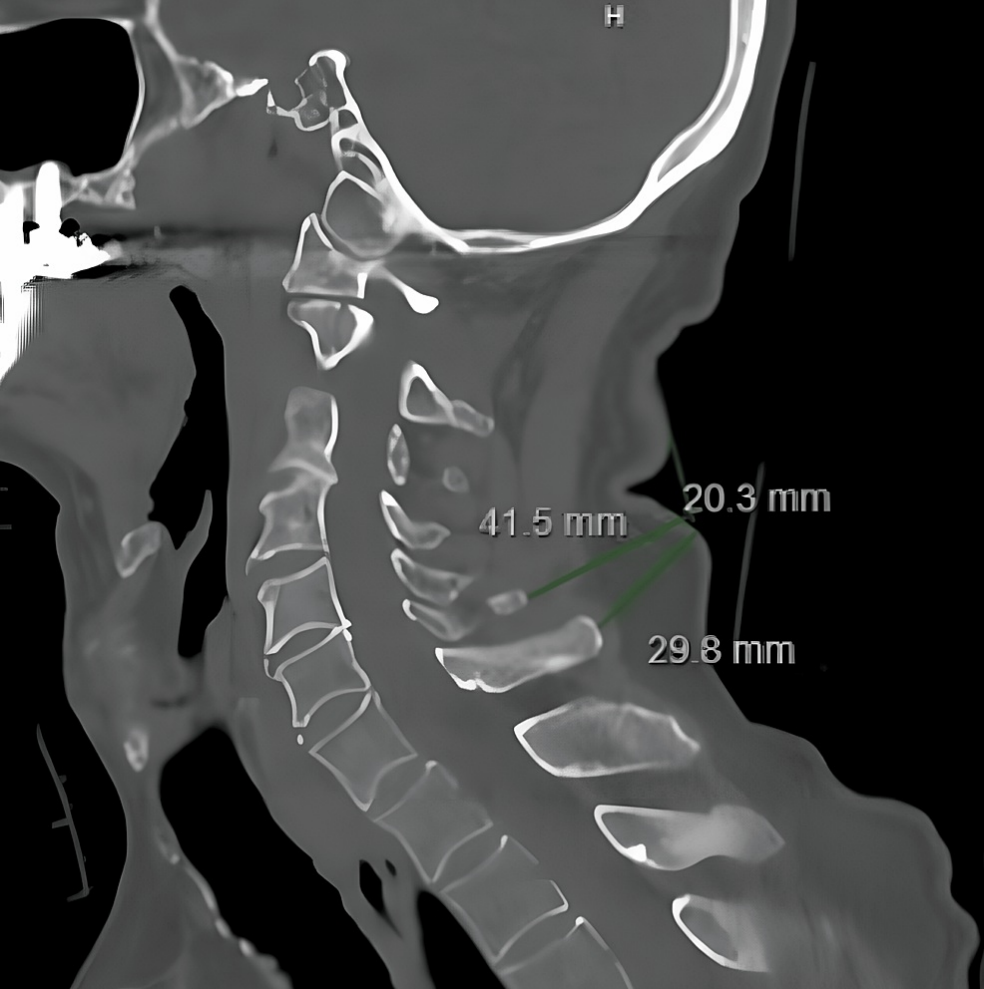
Representative sagittal cervical CT scan showing measurements in a patient with a large posterior skin fold
